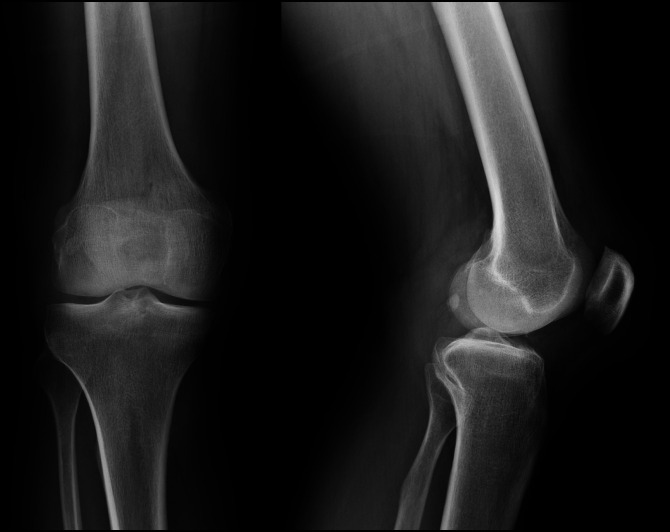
AP (left) and lateral (right) radiographs showing the patient's right knee before the development of his initial skin lesion over the right knee joint.
